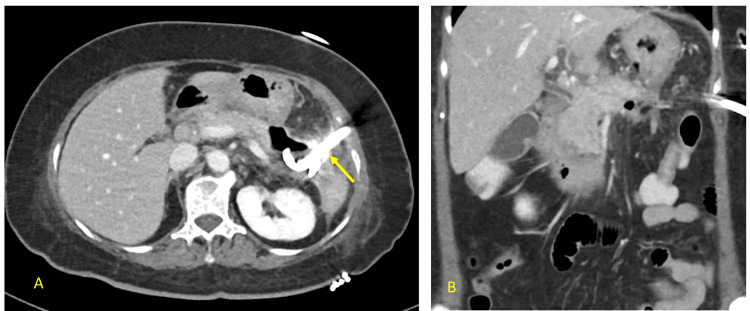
CT scan of the abdomen with contrast shows metallic clip and almost complete resolution of the collection A: Axial section shows the metallic clip (yellow arrow). B: Coronal section shows almost complete resolution of the previously seen collection.
